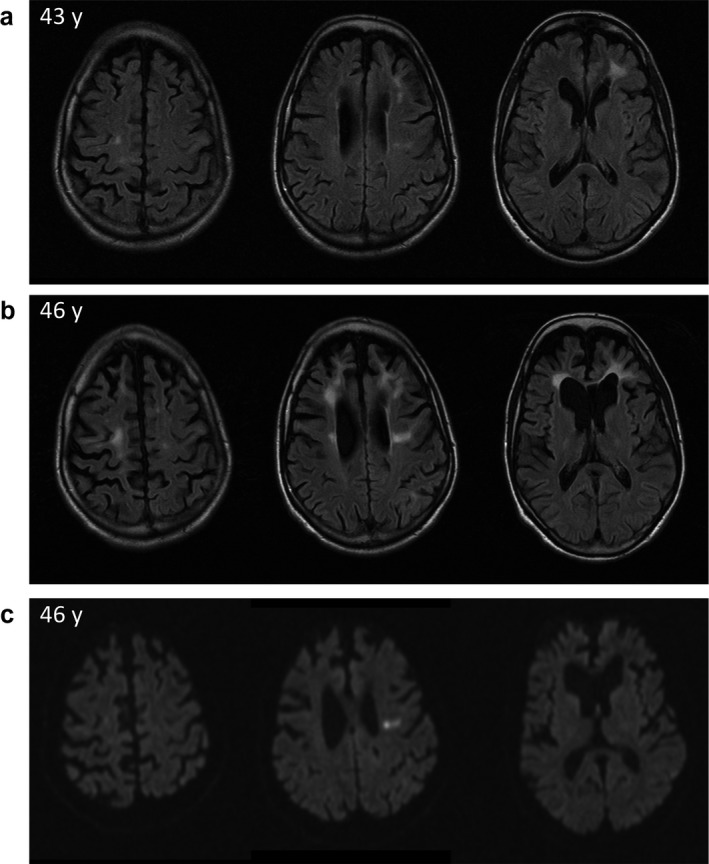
Magnetic resonance imaging (MRI) of the brain. (a) Initial diffuse white matter lesions and gliosis at the age of 43 years. (b) Follow‐up MRI at age of 46 years showing increasing diffuse white matter lesions and progression of frontotemporal cerebral atrophy. (c) Diffusion weighted imaging (DWI) of the follow‐up examination revealing various isolated spots of hyperintense signal as sign of diffusion restriction and characteristic imaging feature for HDLS
